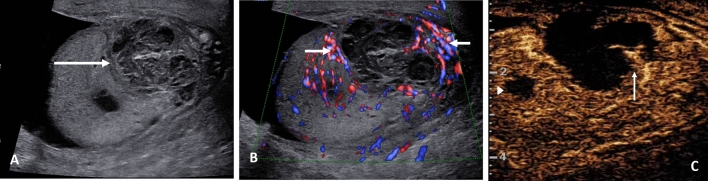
Focal abscess in the right testis in a 42 year old, following severe epididymitis. A Oblique view on B-mode ultrasound demonstrates a heterogenous focal lesion in the inferior aspect of the right testis (arrow). B Color Doppler ultrasound demonstrates an avascular area with some increased flow around the peripheral aspect of the lesion (short arrows). C Contrast enhanced ultrasound demonstrates the abscess cavity as an avascular area, with enhancement of a septation (long arrow). There is a second pocket of abscess formation demonstrated (arrowhead)
